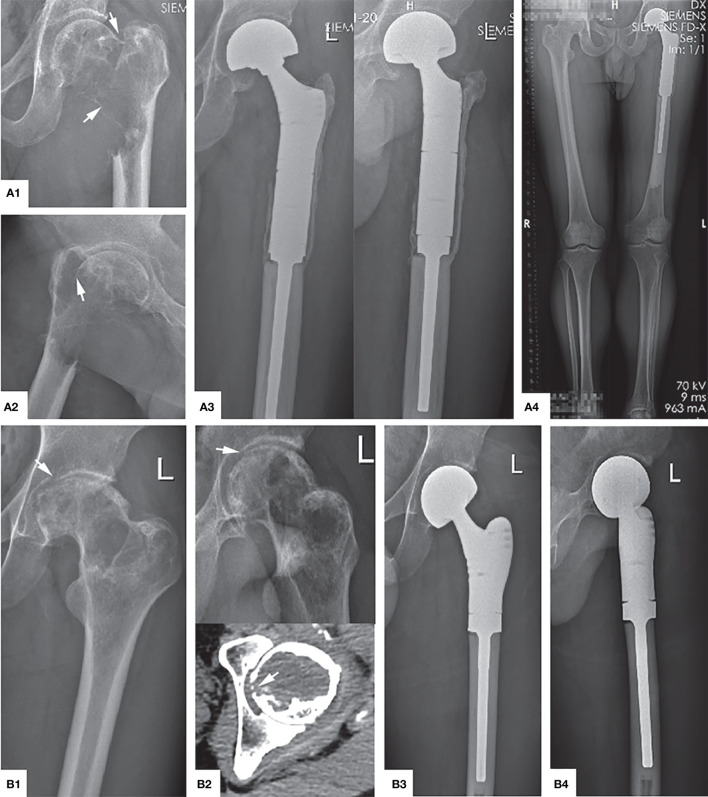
Main indications and typical preoperative and postoperative manifestations of SR. (A1, A2) The tumor has completely eroded the proximal femur, and a significantly displaced pathological fracture has occurred (arrow), which is Campanacci grade III. (A3, A4) Anteroposterior, lateral, and full-length of both lower limbs radiographs showed that the position of the prosthesis was adequate, there was no transparent band around, and the force line was normal 4 years after operation. (B1) The femoral head is compressed to flat due to osteolysis destruction (arrow). (B2) The articular surface was also damaged and ruptured (arrow). (B3, B4) The X-ray shows the contraposition and alignment of the artificial joint prosthesis were satisfactory 41 months after operation.
